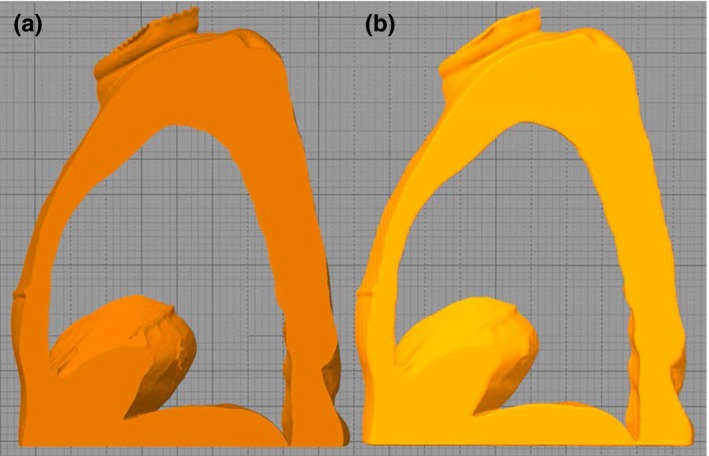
3D‐rendered models of slice number 8. (a) The planning model used to print slice 8, and (b) the model based on the CT scan of the actual printed slice. Figure 3(d) is a photograph of this same slice.
